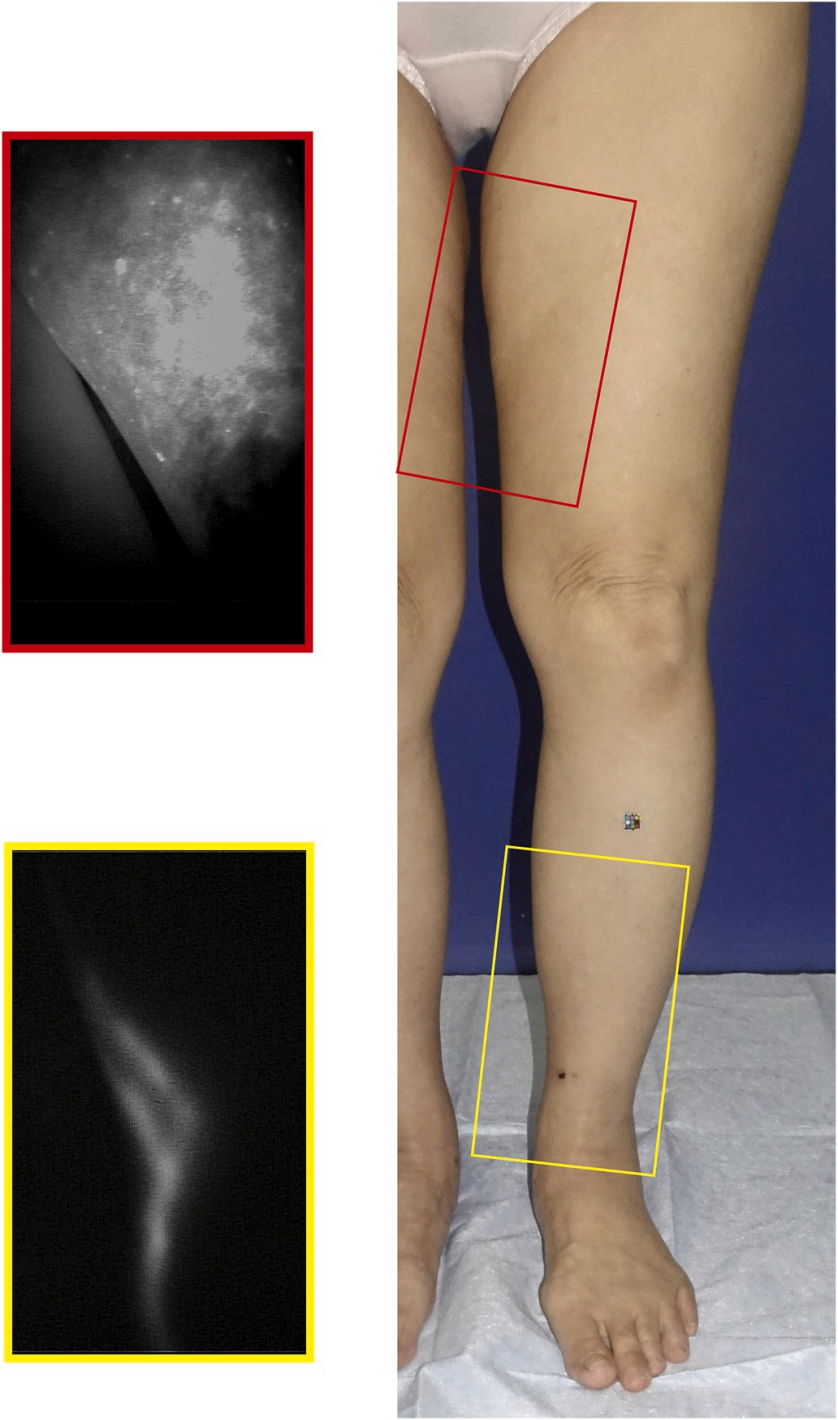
ICG fluorescent lymphograms can be categorized to the region of linear pattern, the region of dermal back flow pattern, and the region where any fluorescent image cannot be seen at all. (A): Linear pattern, (B): Dermal back flow pattern.ICG, indocyanine green. (For interpretation of the references to colour in this figure legend, the reader is referred to the Web version of this article.)3)^99m^Tc lymphoscintigraphy using the injection solution of a radiopharmaceutical agent, standard human serum albumin diethylenetriamine pentaacetic acid technetium (^99m^Tc) (Poolscinti® Injectable), should be conducted in all patients who were included in the primary registration within 8 weeks after ICG fluorescent lymphography in order to determine the sensitivity and specificity of lymphoedema diagnosis by ICG fluorescent lymphography when considering ^99m^Tc lymphoscintigraphy as one of the standard diagnostic methods. ^99m^Tc lymphoscintigraphy should be conducted at 120 min after radioisotope administration.
